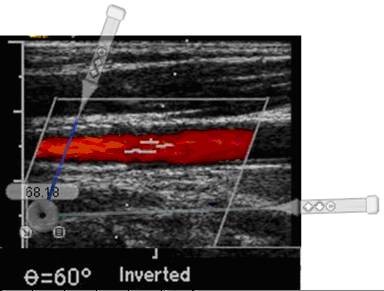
Doppler angle cursor misaligned with artery axis. Angle remeasured with "Screen Protractor" [30]. The protractor is moved over the image to align one protractor cursor with the Doppler beam cursor and the other protractor cursor with the artery axis. Here the protractor has been moved aside to allow easy visualization of the original image. The proximal end of the stent is visible in the left half of this image.
